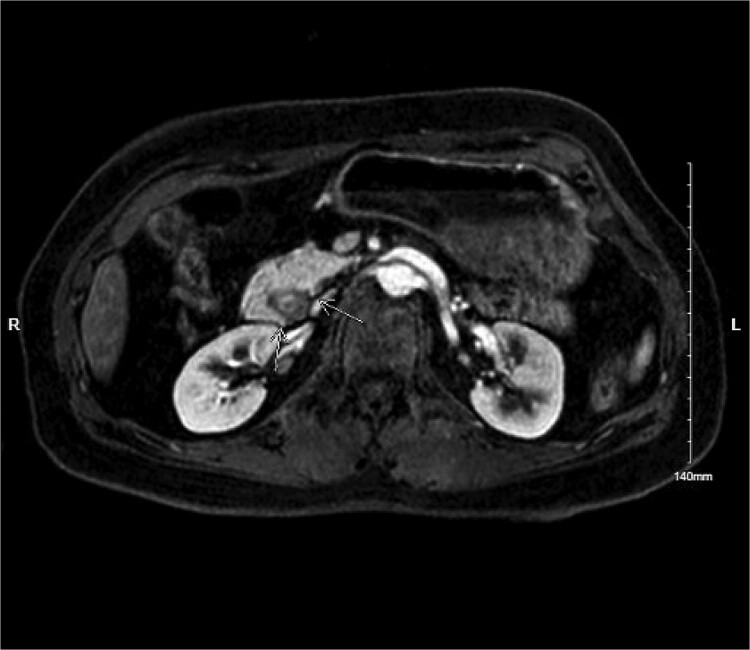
MRI MRCP showing annular pancreas causing near complete obstruction of the second portion of the duodenum.
